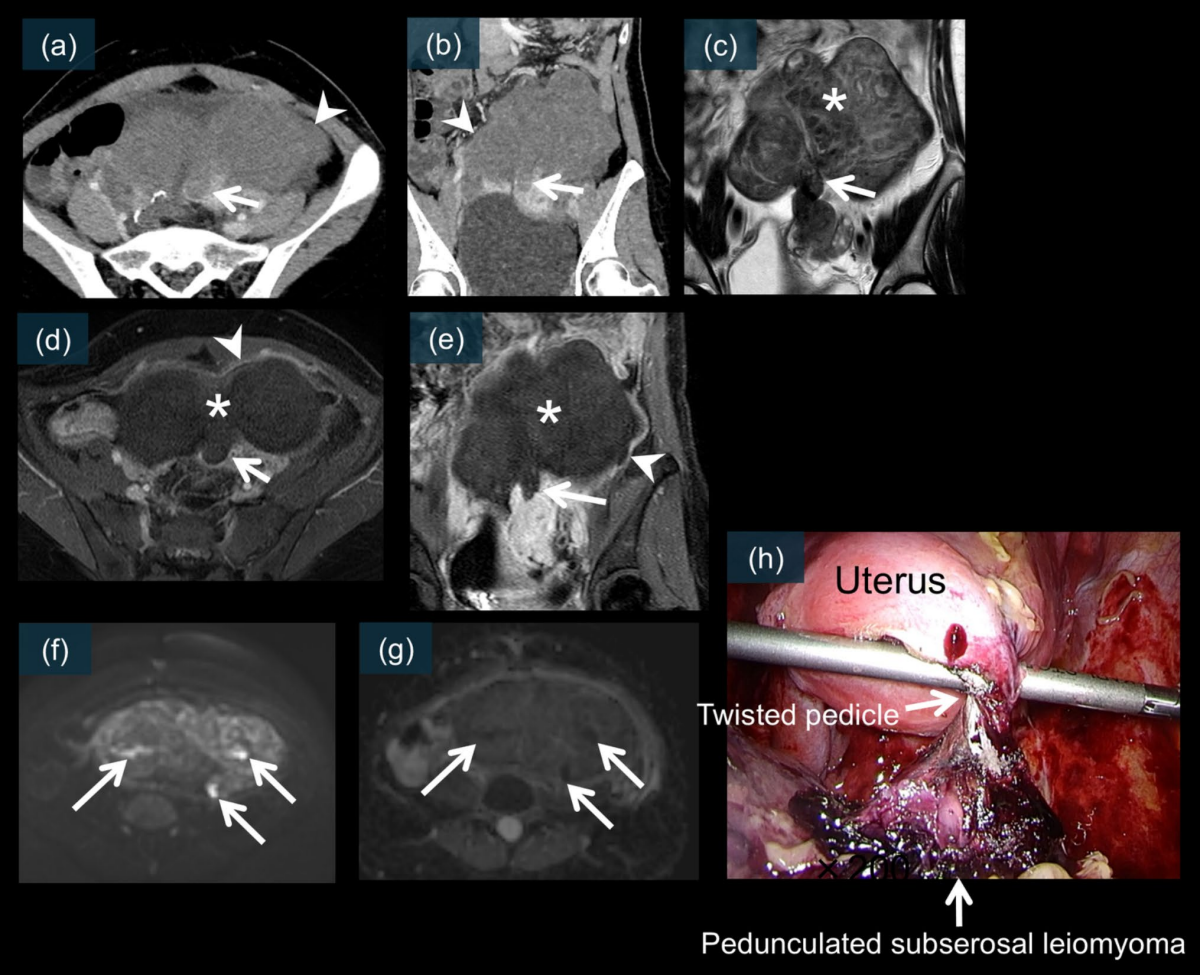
Contrast-enhanced CT images of a 35-year-old patient. CT images (a and b) show a 1.5 cm pelvic mass connected to the uterus by a stalk. The mass demonstrates poor internal enhancement with a thin peripheral rim of enhancement (white arrowheads). A low contrast enhancement area is observed in the uterine region adjacent to the mass, which is called the “dark fan sign (white arrows).” This CT finding is believed to reflect the decreased perfusion of the uterine muscle adjacent to the subserosal leiomyoma with acute torsion. On T2WI (c), the stalk connecting to the uterus is clearly depicted and appears slightly enlarged (white arrow). The mass shows a heterogeneous internal signal intensity (white asterisk). On contrast-enhanced MRI (d and e), neither the non-enhancing wedge-shaped area (white arrows) corresponding to the “dark fan” sign nor the mass itself shows enhancement (white asterisks). A thin enhanced rim is observed at the periphery (white arrowheads). The nonenhanced area corresponds to necrosis, and the usual rim of enhancement corresponds to obstructed peripheral veins with edema. DWI/ADC (f and g) shows partial diffusion restriction within the mass, corresponding to ischemia and hemorrhagic necrosis (white arrows). (h) is a laparoscopic image showing a subserosal leiomyoma that has undergone a 480° torsion
